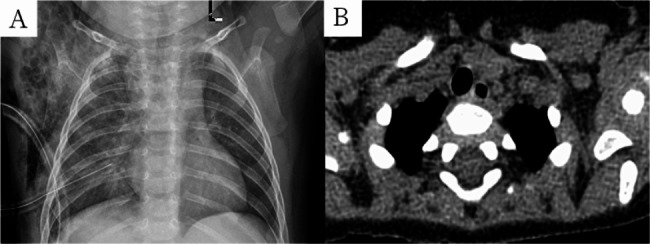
Recovery status of children with subcutaneous emphysema after surgery: (3 A) Chest X-ray showing subcutaneous emphysema on the right side 3 d after surgery; (3B) One month after surgery, chest CT showing the disappearance of subcutaneous emphysema on the right side
